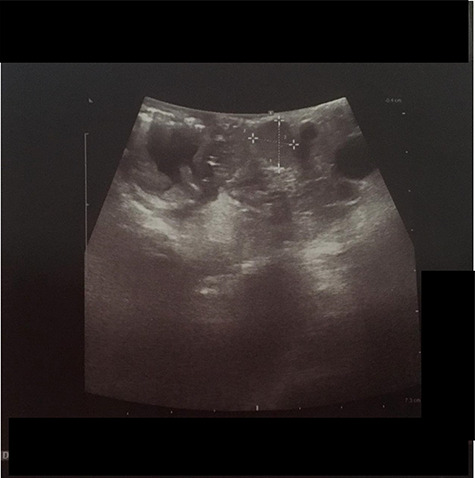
IOUS (intraoperative ultrasound) performed during surgery shown hypoechogenic lesion in the head of pancreas (between white marks).
